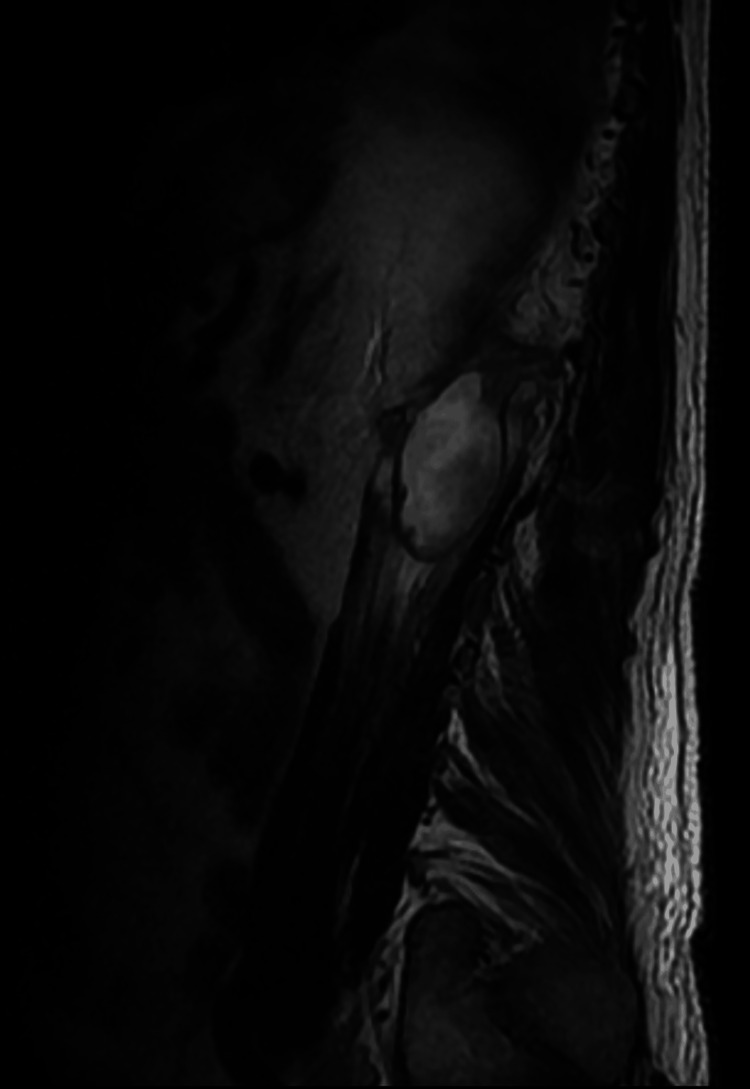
FLAIR MRI. Collection at the level of the psoas from T12 to L1 with well-defined borders and homogeneous content, confirming the presence of an abscess FLAIR: fluid-attenuated inversion recovery
